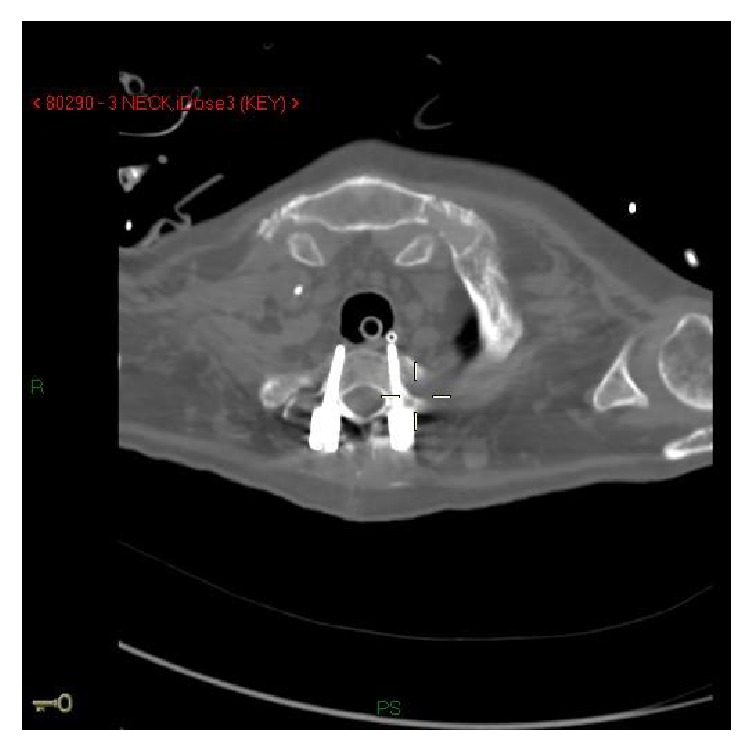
Computed tomography (axial view): fixation screw abetting the esophagus.
